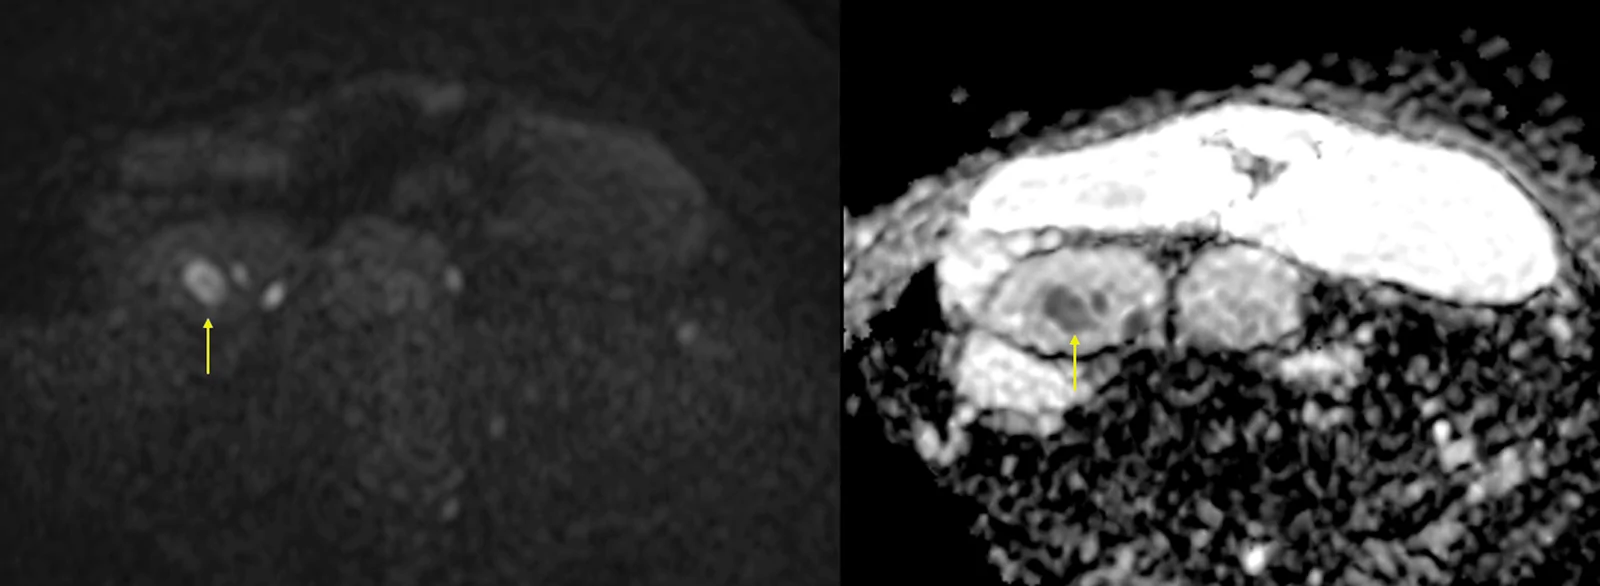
Pelvic MRI: DWI and ADC map There are foci of proton diffusion restriction in dependent areas of the lesions due to the presence of concentrated blood products (yellow arrows). MRI: magnetic resonance imaging; DWI: diffusion-weighted imaging; ADC: apparent diffusion coefficient
